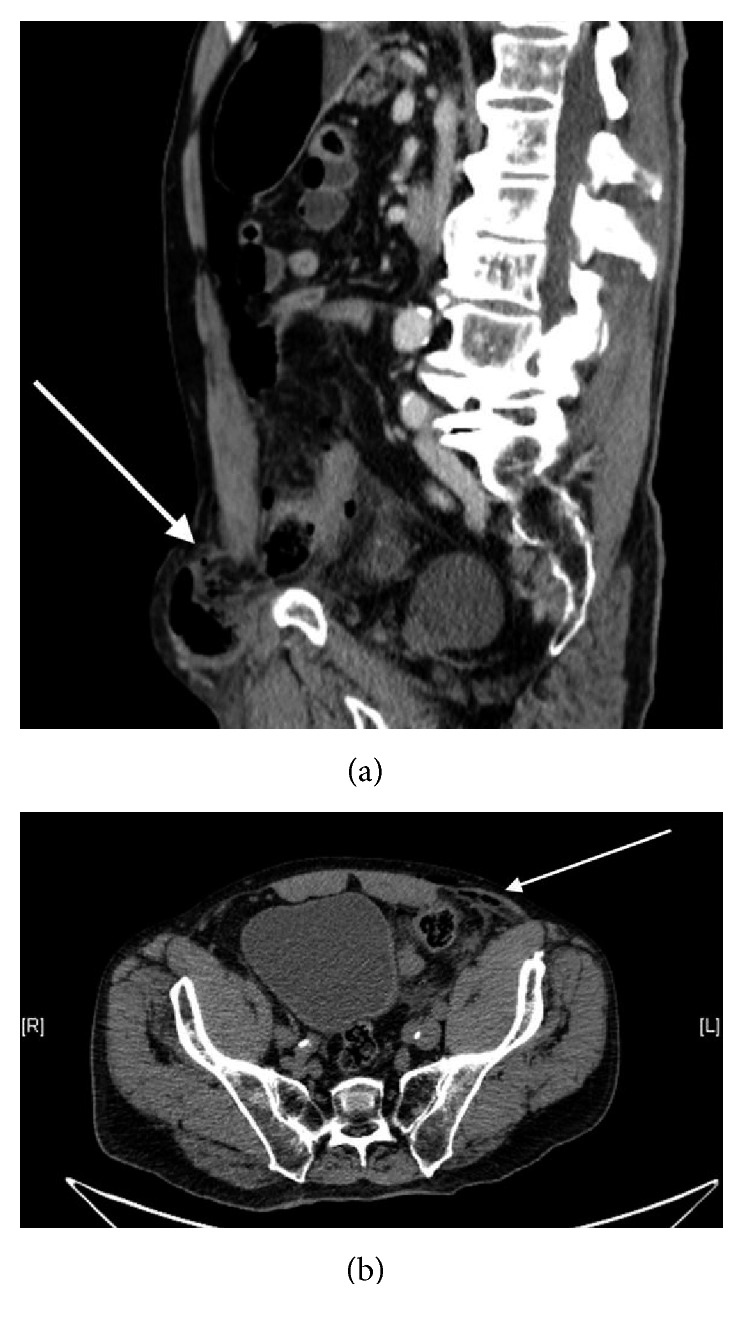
Femoral hernia with free air in preoperative CT: (a) sagittal and (b) axial.
